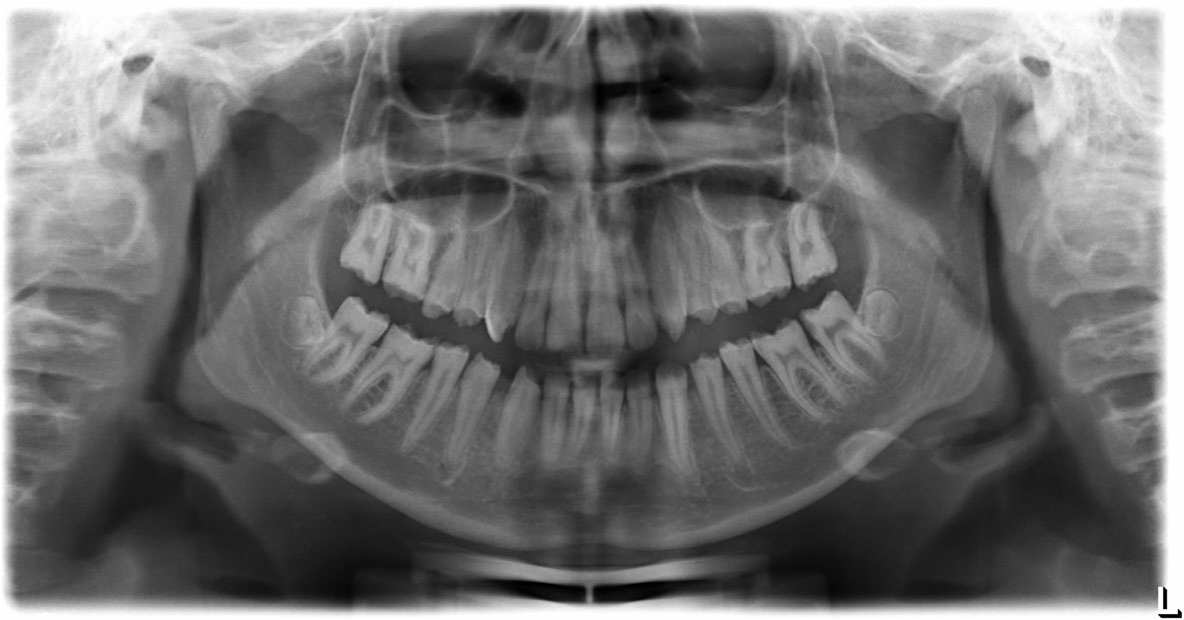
Orthopantomogram prior to treatment
